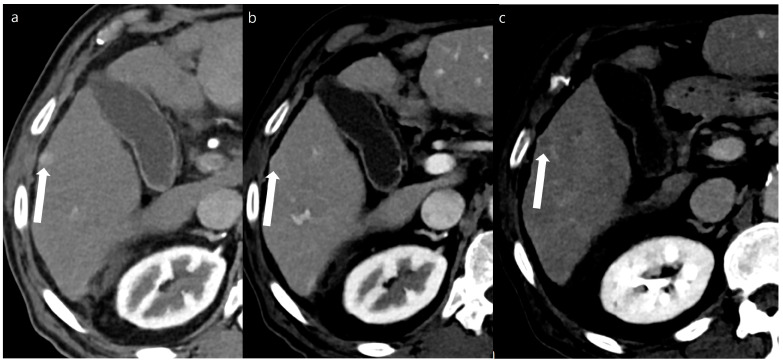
A 65-year-old man with a 0.8 cm sized hepatocellular carcinoma at segment 5 of the liver (arrows). Arterial phase (a), portal phase (b), and delayed phase (c) images of a double low-dose CT using a deep-learning-based contrast-boosting model show a well-enhancing nodule on the arterial phase (a), with washout on the portal phase (b) and the delayed phase (c).
